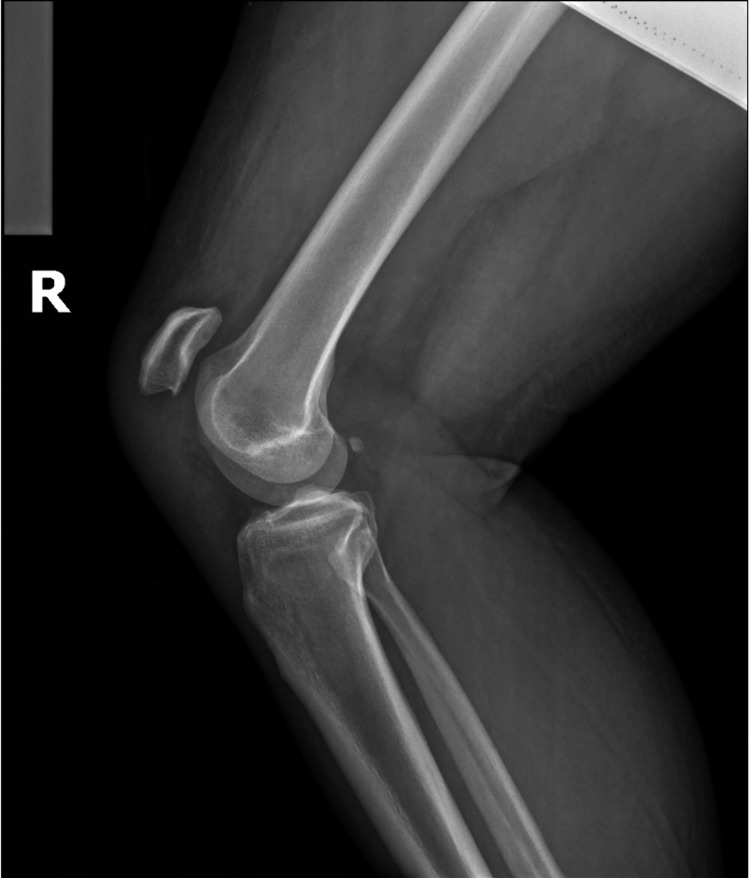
Lateral preoperative radiograph of the right knee Lateral view of the right knee obtiained at presentation demonstrating patella alta, consistent with complete rupture of the patellar tendon. Superior displacement of the patella correlates with the patient’s clinical inability to extend the right knee.
